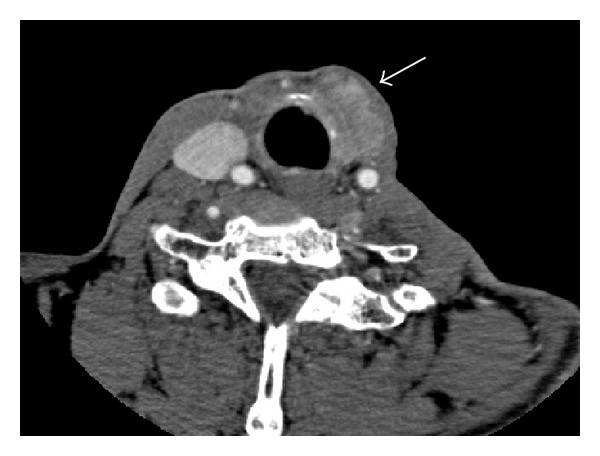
CT scan showing cricoid cartilage invasion by thyroid carcinoma (arrow).
